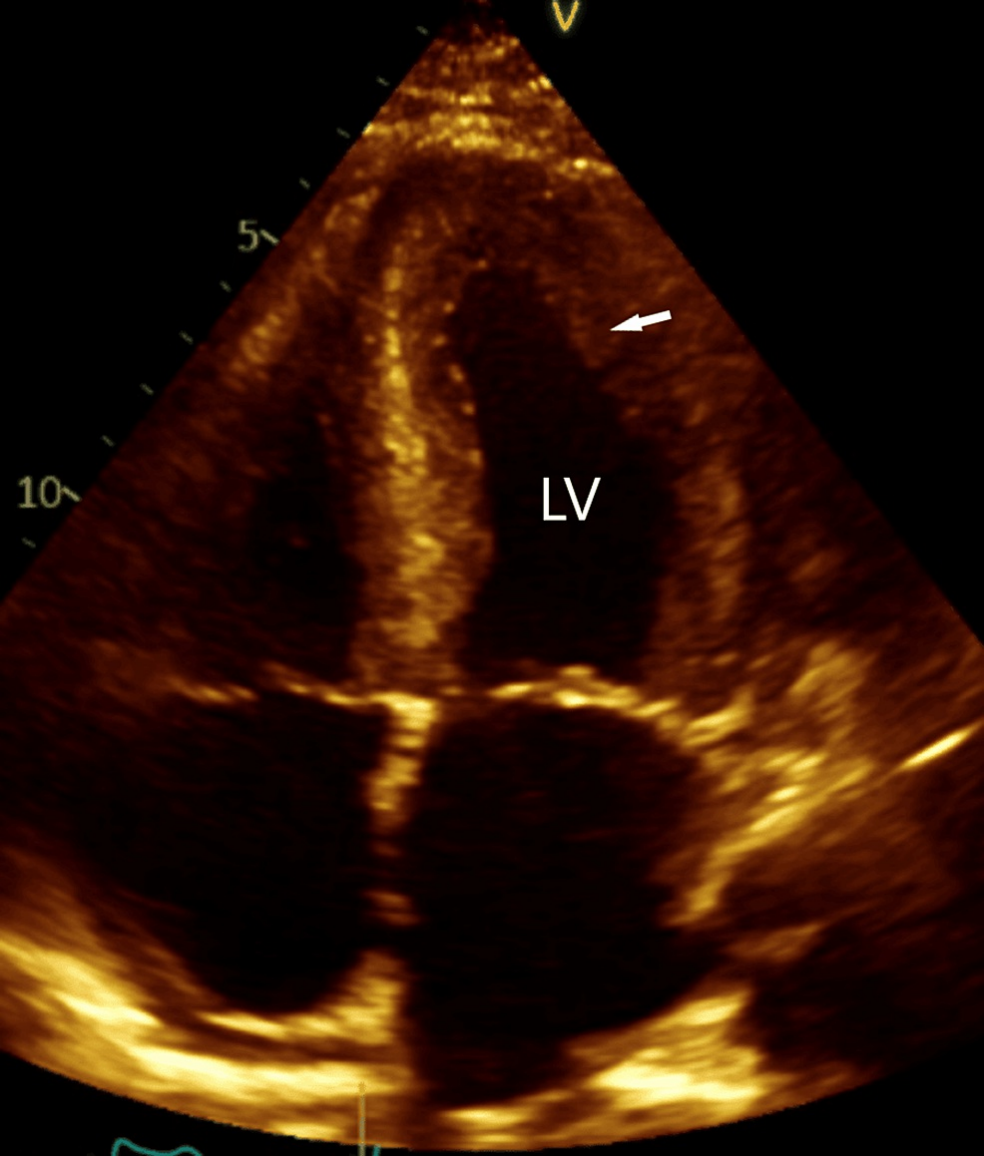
Four-chamber echocardiogram view. Four-chamber echocardiogram view showing Increased left ventricular wall thickness (arrow). LV: left ventricle
